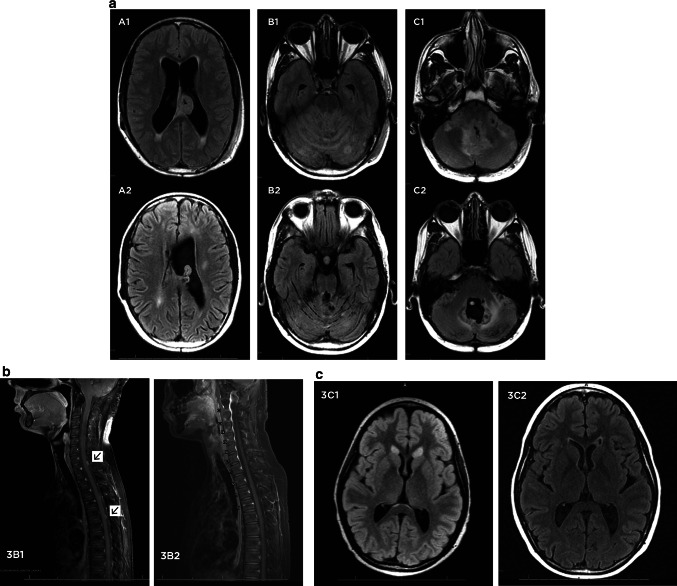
MRI scans demonstrating treatment response in patients 1 and 4. a Fluid attenuated inversion recovery (FLAIR) MRI scans in patient 1 at baseline (*top row*, images *A1*, *B1*, and *C1*) and after 15 cycles of intraventricular methotrexate (*bottom row*, figures *A2*, *B2*, and *C2*), 10 months after initiation of the first cycle. There has been a considerable decrease in disease burden in the lateral ventricles, cerebellar folia, fourth ventricle, and cerebellopontine angles. FLAIR sequences are demonstrated because the majority of the tumor burden in the brain was non-enhancing and best visualized on FLAIR. b T1-weighted MRI of the spine with gadolinium in patient 4 at baseline (*left*, image *3B1*) and after 15 cycles of intraventricular methotrexate (*right*, image *3B2*). Two small metastatic spine lesions (demonstrated by *arrows*) have disappeared. c Axial FLAIR sequences in patient 5 at baseline (image *3C1*) and after 18 cycles (image *3C2*) of intraventricular methotrexate. FLAIR sequences are demonstrated because both were non-enhancing lesions best visualized on FLAIR. There has been a considerable decrease in the lesions, both of which have nearly resolved
